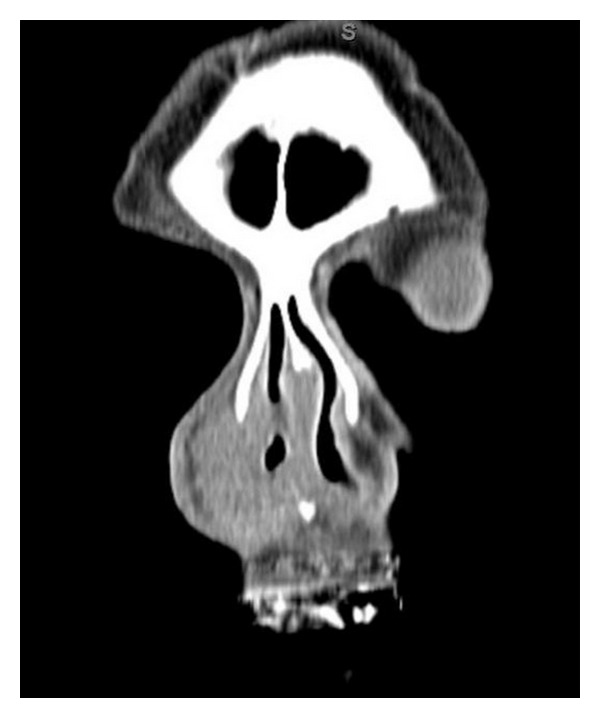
Coronal contrast enhanced CT scan showing large subcutaneous soft tissue density lesion of the nasal ala.
